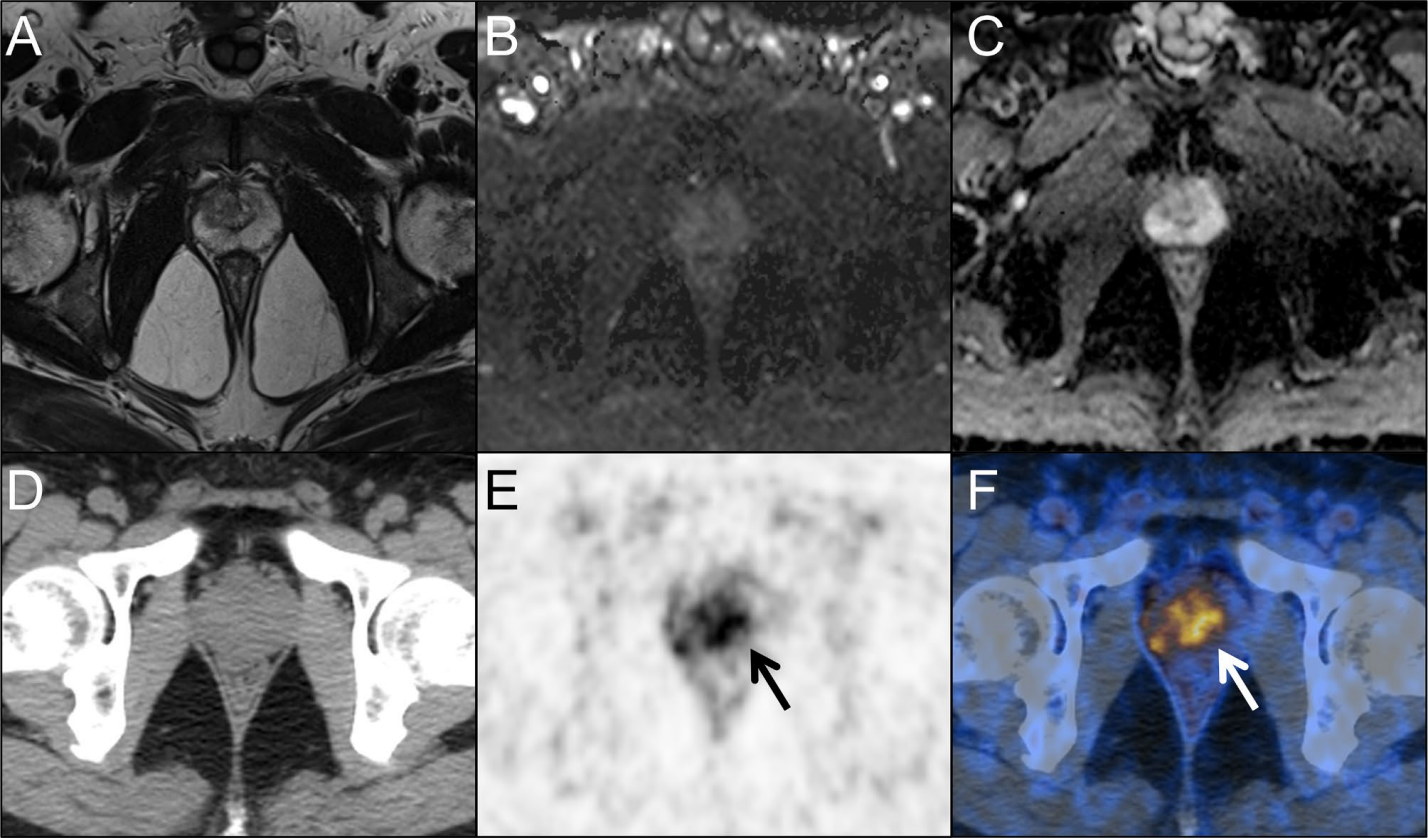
A 55-year-old man presented with a persistently elevated prostate-specific (PSA) range of 4.37–6.82 ng/ml over a period of one year. Magnetic resonance imaging (MRI) (A, T2-weight imaging; B, DWI imaging; C, ADC map) didn’t revealed the presence of any malignant lesions. Positron emission tomography/computed tomography (PET/CT) images (D, CT; E, PET; F, fusion) showed intensive prostate-specific membrane antigen (PSMA) uptake in the center gland and right periphery of the prostate (arrows). According to the predictive model, the probability of clinically significant prostate cancer for this lesion is 0.67 (> 0.35). Subsequent prostate biopsy result in a Gleason score 4 + 3 prostate cancer
